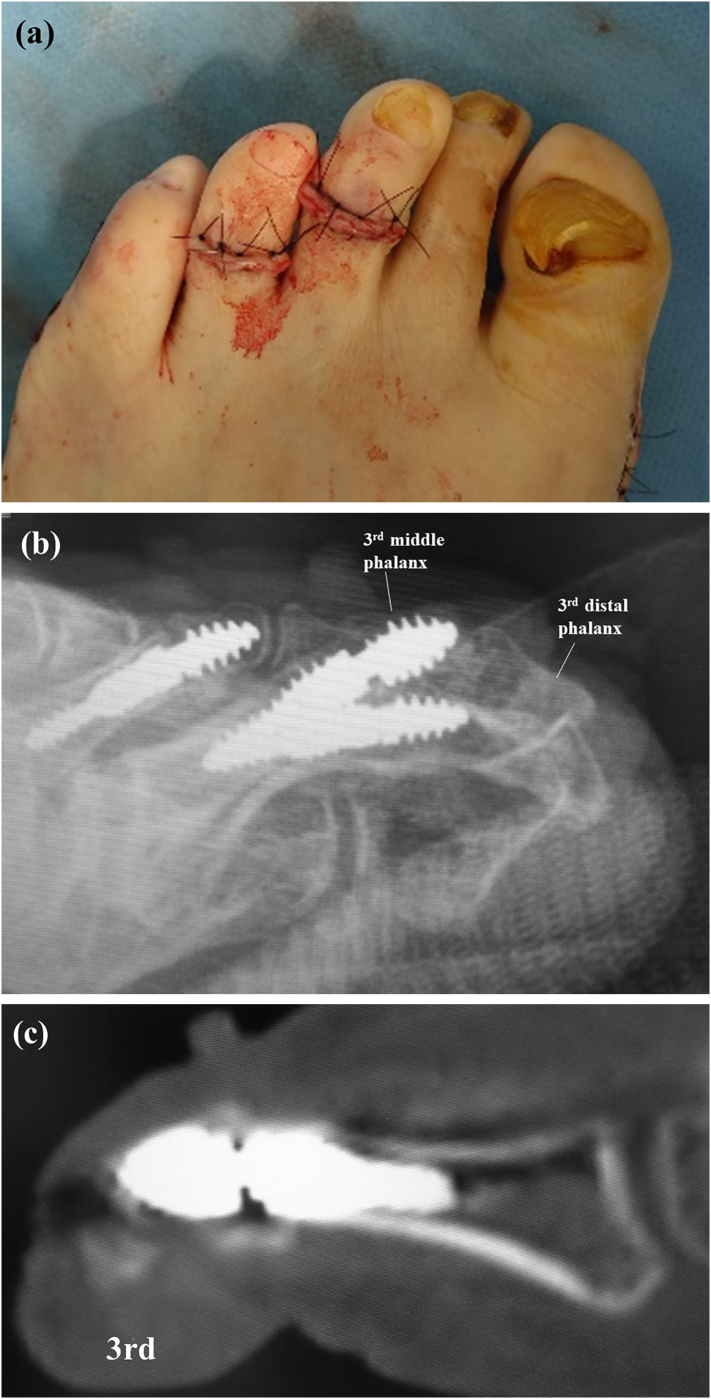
Intraoperative photograph showing correction of the hammer deformity of the third toe (a). A lateral radiograph (b) and sagittal computed tomography image (c) obtained soon after surgery confirm that the implant is correctly positioned.
